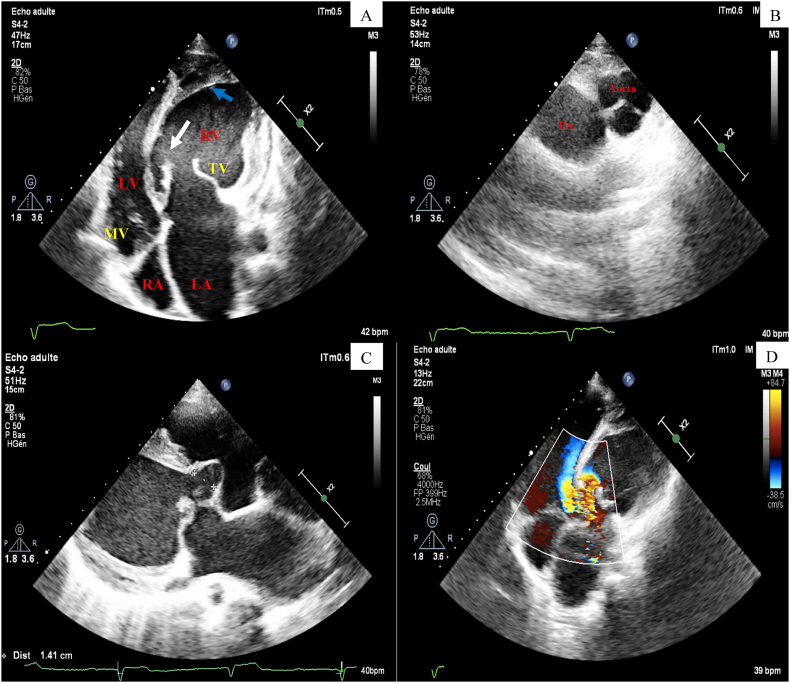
TEE findings: (A) Apical four chamber view showing the aspect of double discordance. Note the septal insertion of the left atrioventricular (AV) valve (white arrow) which is slightly apical compared to the right AV. The moderator band (blue arrow) can also be seen in the left sided ventricle. (B) Short axis view showing the anterior and left location of the aortic valve. (C) Modified parasternal long axis view showing a large perimembranous ventricular septal defect closed by spontaneously by a septal aneurysm. (D) Apical four chamber view showing tricuspid regurgitation that was quantified as moderate regurgitation and jet from the left sided ventricle to the right sided ventricle. (For interpretation of the references to colour in this figure legend, the reader is referred to the Web version of this article.)
